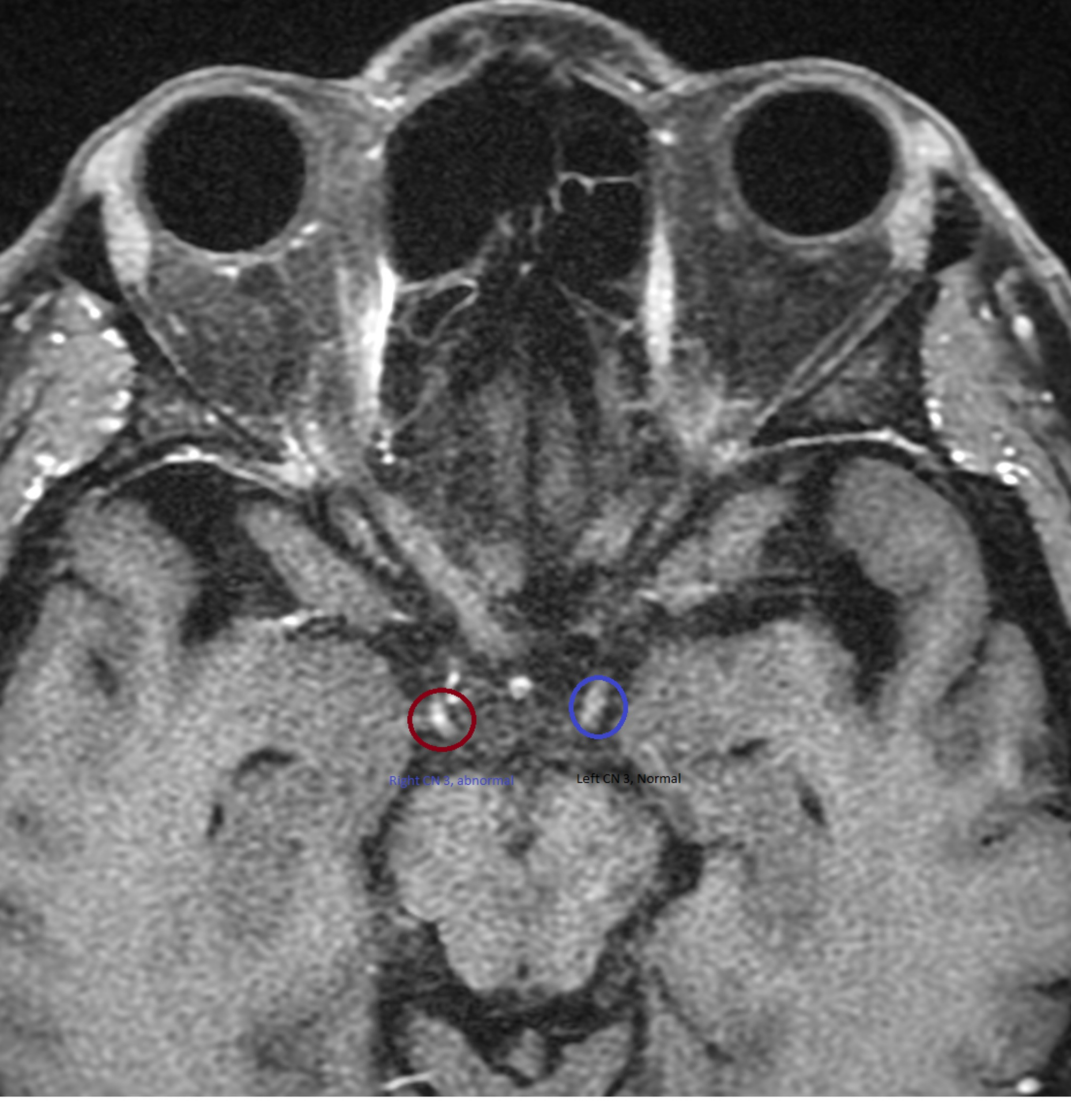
MRI Head T1, Axial Section. In the figure the red circle shows abnormal enhancement of right CN 3 and blue circle shows normal left CN 3 CN: cranial nerve
